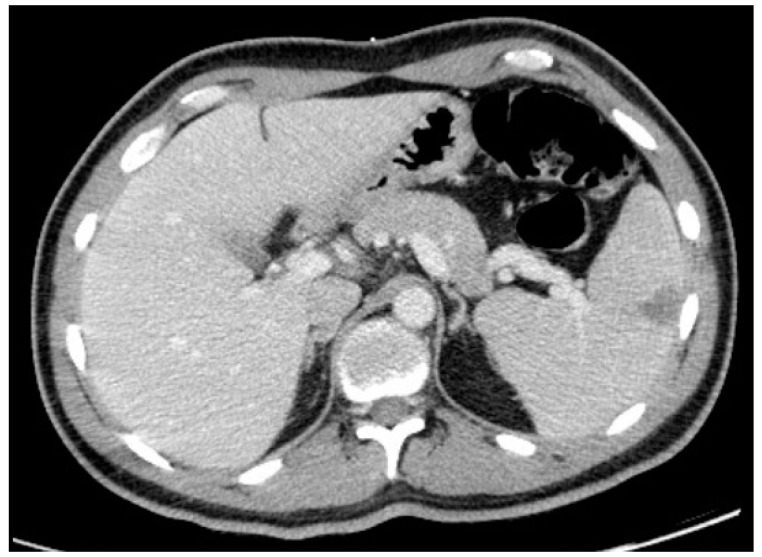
Thoraco-abdominopelvic CT showing splenic infarction.
